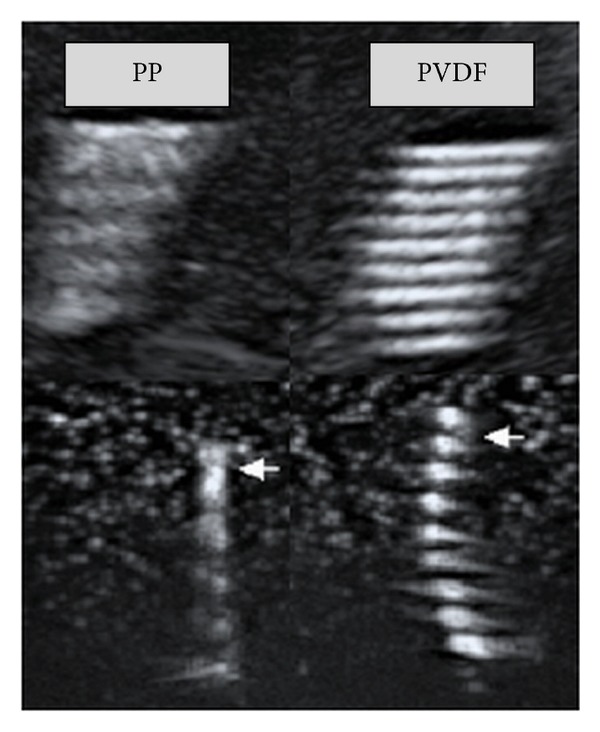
Sonography of both kinds of slings embedded in gelantine, performed to compare their individual sonographic visibility. Upper row: longitudinal aspect; lower row: cross-sectional aspect.
